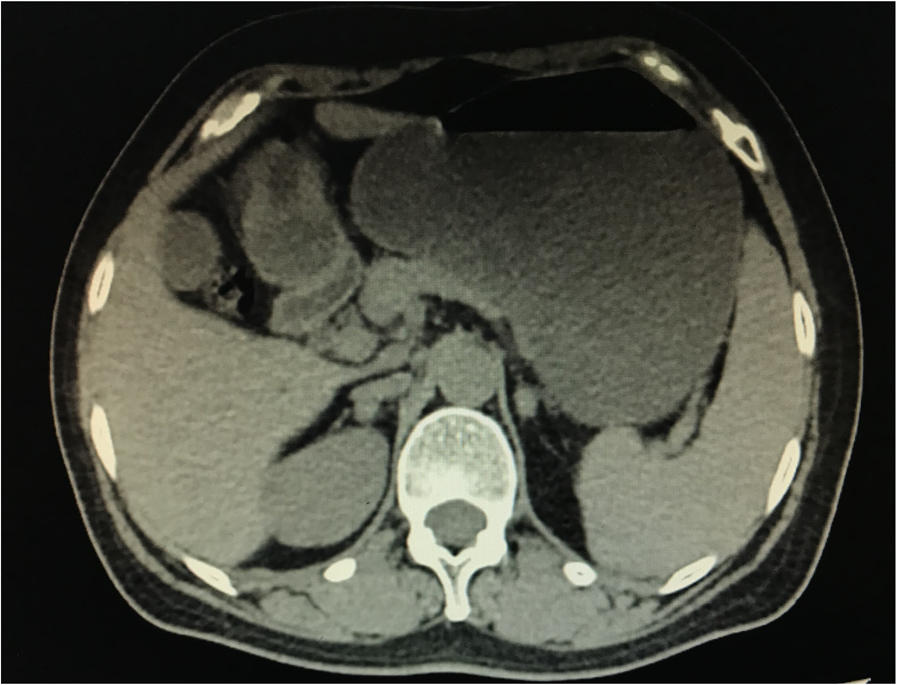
CT scan (9 months after discharge) revealed a continuously shrunken adrenal mass, measured 1.4 × 0.9 cm on the left and 1.3 × 0.9 cm on the right, compared with 9 months ago
